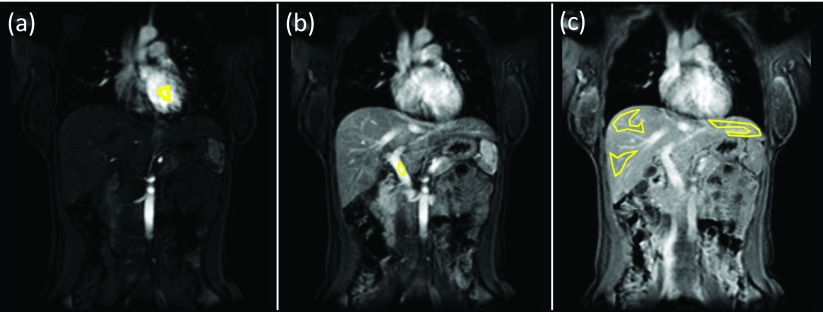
Example of ROI placement for DCE MRI quantification. Intra-ventricular ROI placement for AIF (a), PV ROI placement of PVIF (b) and parenchymal ROI placement (c) for segments II/III (far left), segments V/VI (right lower) and segments VII/VIII (right upper). Parenchymal ROIs were placed in each of the three locations on five slices.
